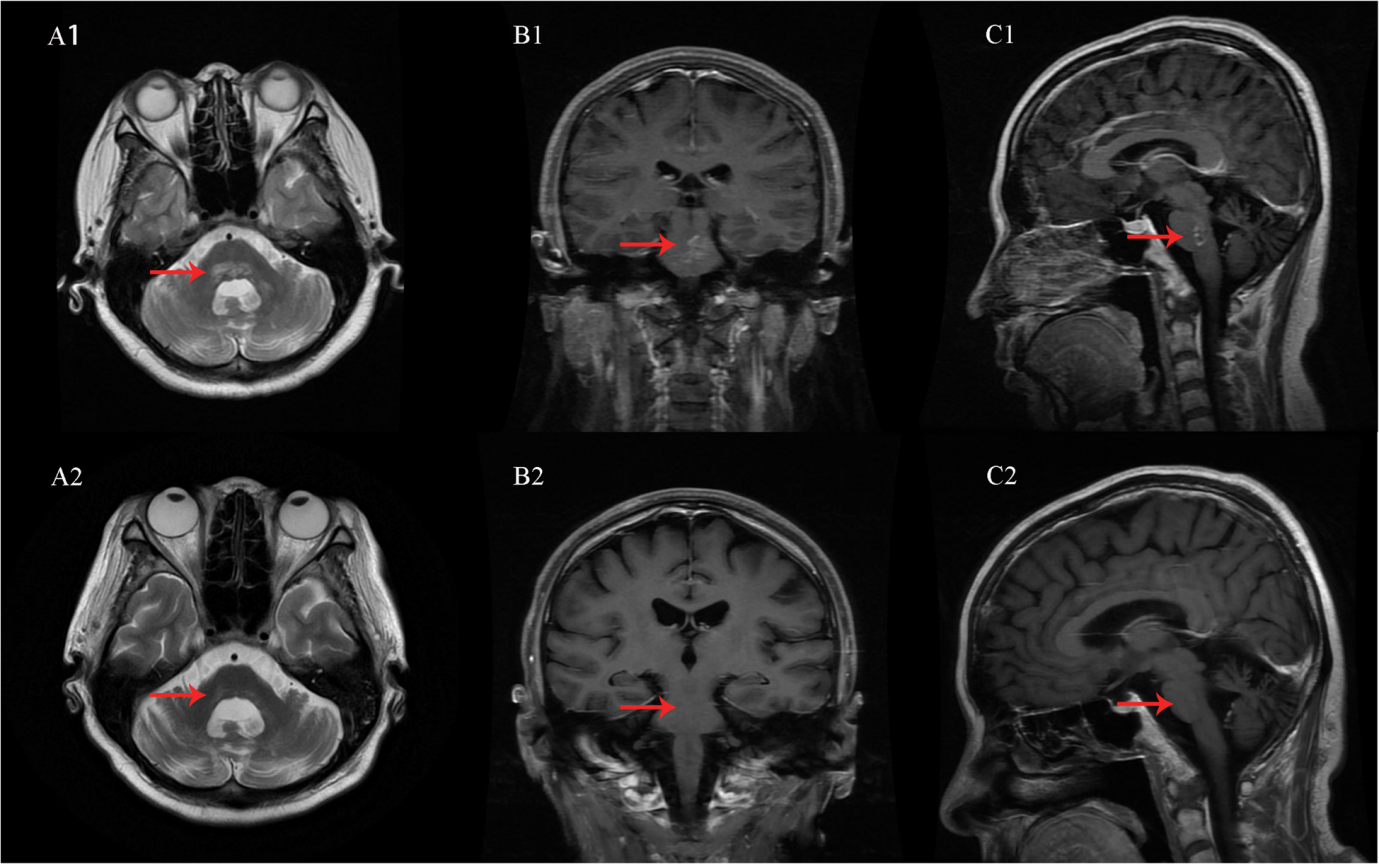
Neuroradiological images on admission and 1 month after steroid therapy. Representative axial T2-weighted imaging (A1, A2), coronal (B1, B2) and sagittal (C1, C2) T1-weighted imaging with gadolinium enhancement on brain MRI demonstrate changes of MRI features after steroid therapy. The images performed on admission show multiple punctuate hyperintense lesions within the pons, brachium pontis, and cerebellum, with a perivascular enhancement pattern (red arrow, top row). A decrease in the number and extent of pathology is observed on a brain MRI after steroid treatment (red arrow, bottom row)
